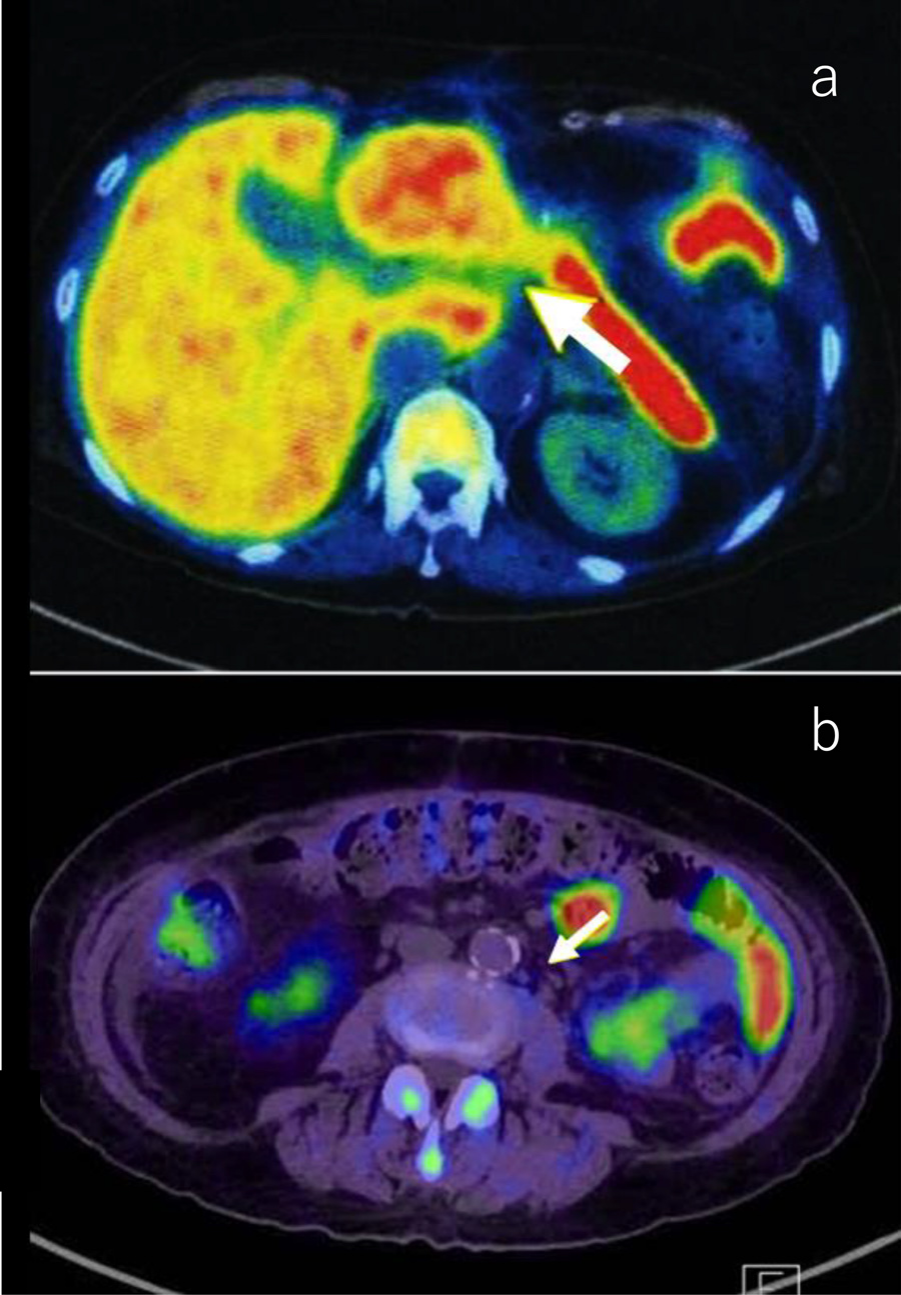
Axial fusion image of MET-PET/CT after the surgery. Lymph nodes around the hepatic artery (A) and around the aorta (B), which showed high accumulation on FDP-PET/CT, show low accumulation on MET-PET/CT (SUV max, 3.7 and 1.3, respectively). The findings are consistent with sarcoidosis. MET-PET/CT, methionine-positron emission tomography/computed tomography.
